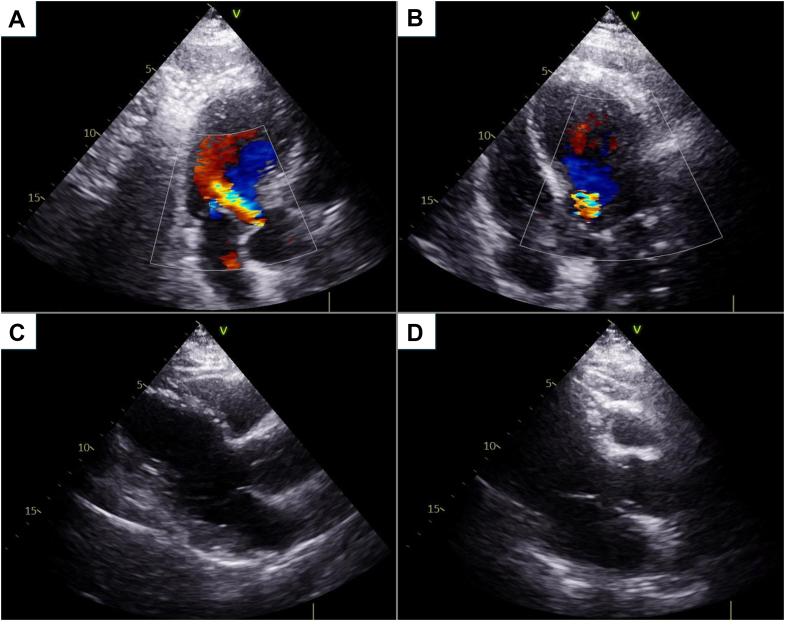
Baseline Echocardiogram Baseline echocardiogram showed grade III/IV AR (A and B) without evidence of an intimal flap (C and D). AR = aortic regurgitation.
